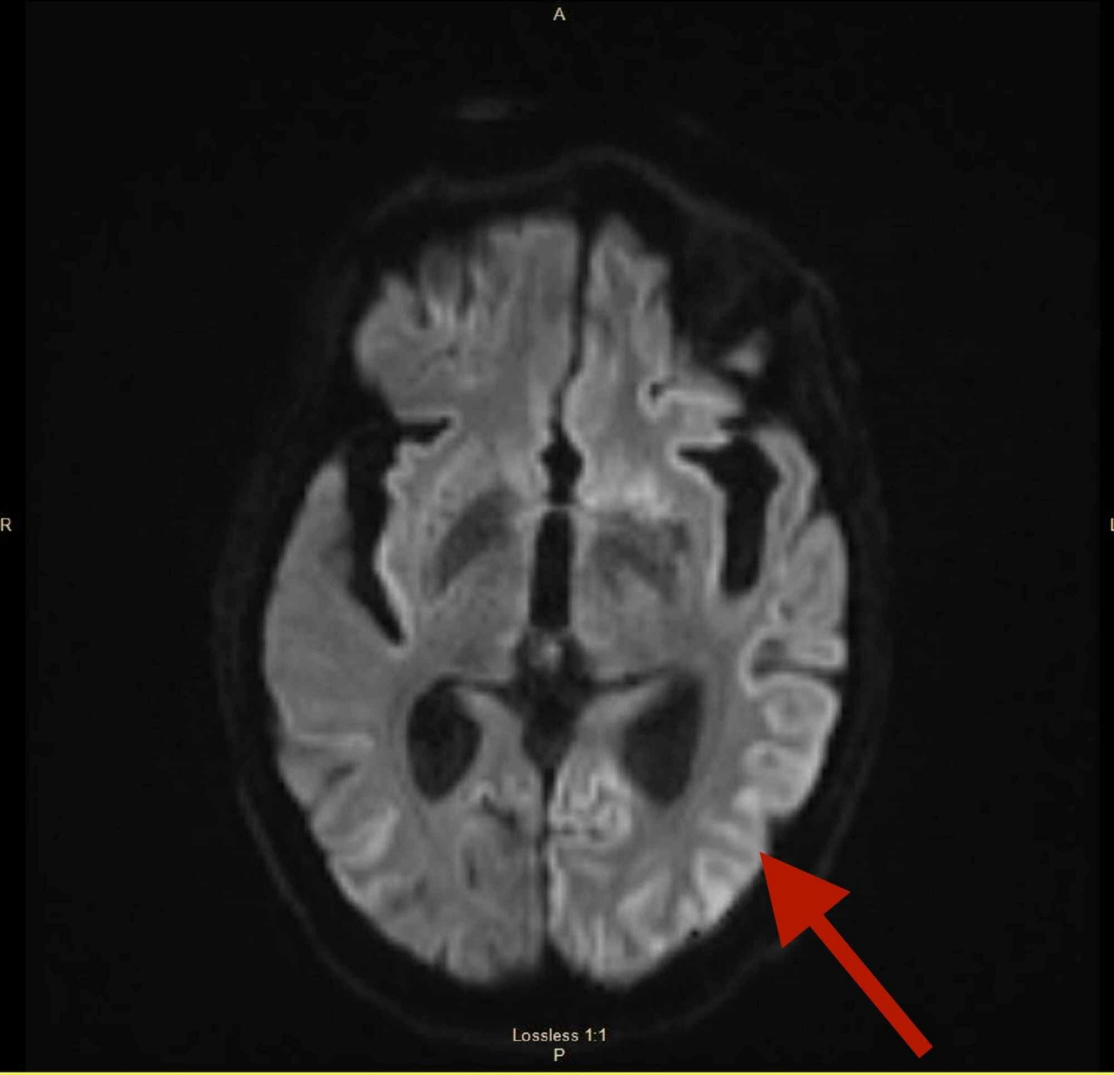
MRI brain: DWI axial image showed the ribbon-like signal hyperintensity of cerebral cortical gyri, which is known as the cortical ribbon sign DWI, diffusion-weighted imaging
